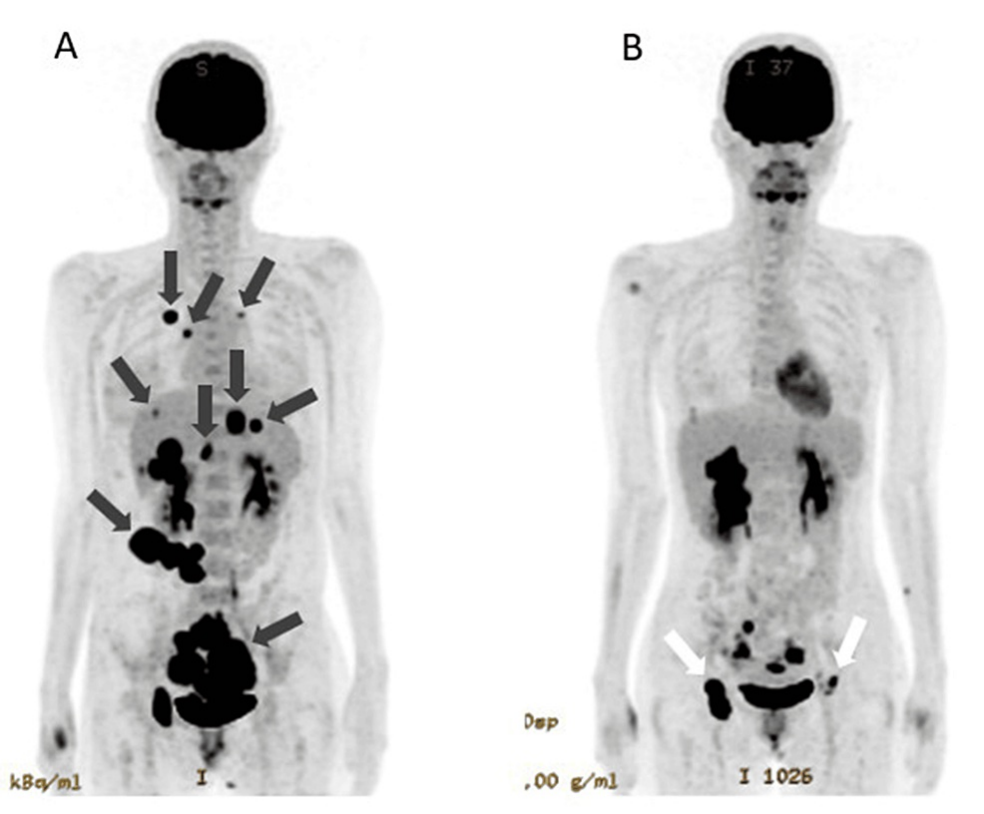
Marked shrinkage of cancer on PET-CT (A) Primary ovarian cancer and multiple metastases to the liver, peritoneum, and lungs are seen (black arrows). (B) PET-CT at 142 days shows marked shrinkage of the primary tumor, disappearance of lung metastases and peritoneal dissemination, and shrinkage of liver metastases. The white arrows indicate inflammatory reaction due to inoculation of dendritic cell vaccine to the inguinal region.
